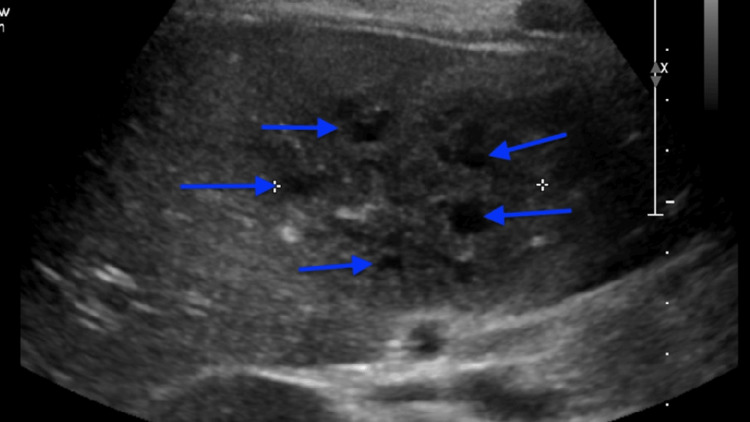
Abdominal ultrasound of liver phlegmon in transverse view, centrally showing multiple hypoechoic phlegmons without coalescence to abscess as denoted by blue arrows
